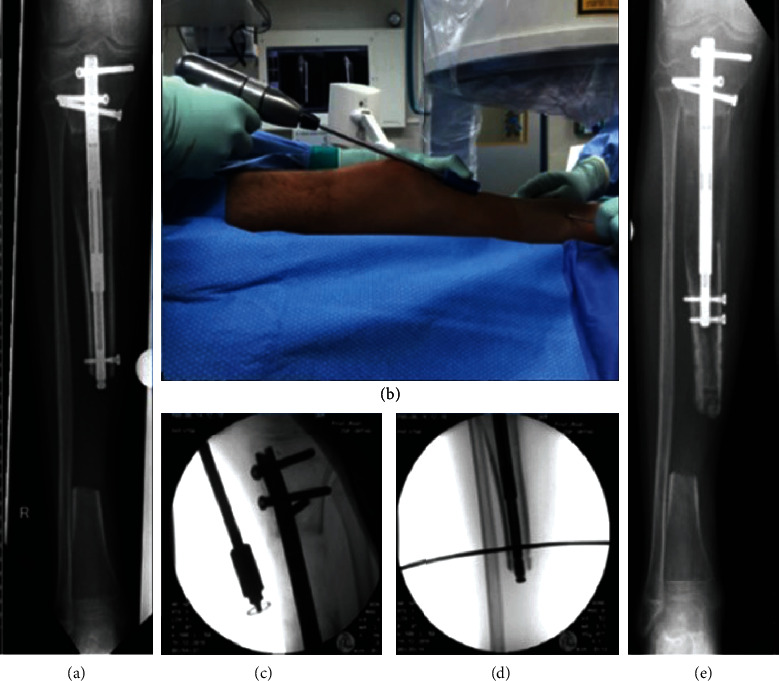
Patient 2. (a) Radiograph showing proceeded bone segment transport. (b–d) Clinical and fluoroscopic presentation of intraoperative retraction of the nail using the fast distractor tool with temporary K-wire transfixation of the transport segment. (e) Radiograph showing advanced bone segment transport.
